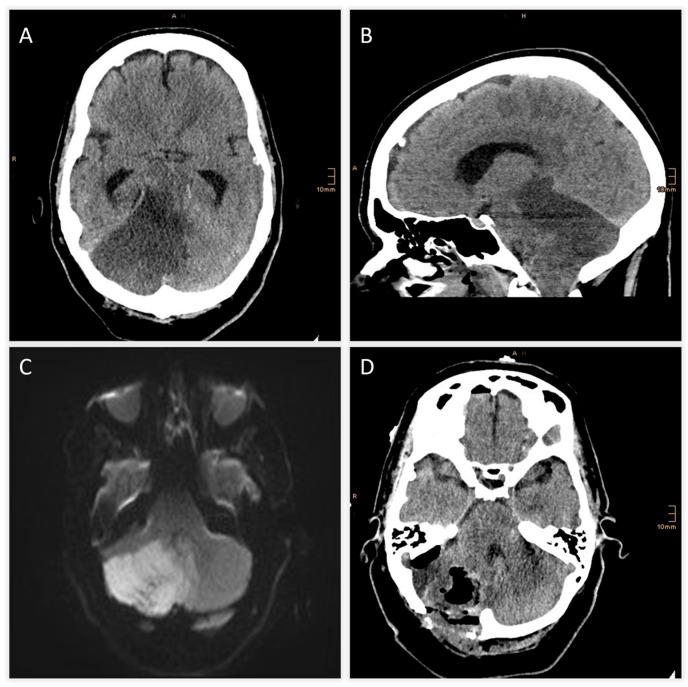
(A,B) Follow-up CT revealing an expanding infarct of the right cerebellum with mass effect and apparent hypodensity in the pons, midbrain and diencephalon, raising suspicion of brainstem infarction. (C) Axial diffusion weighted image obtained after suspicious CT findings excluding brainstem infarction and demonstrating predominantly cerebellar edema. (D) Postoperative CT scan following suboccipital decompression, partial cerebellar tissue resection and ventricular drainage.
